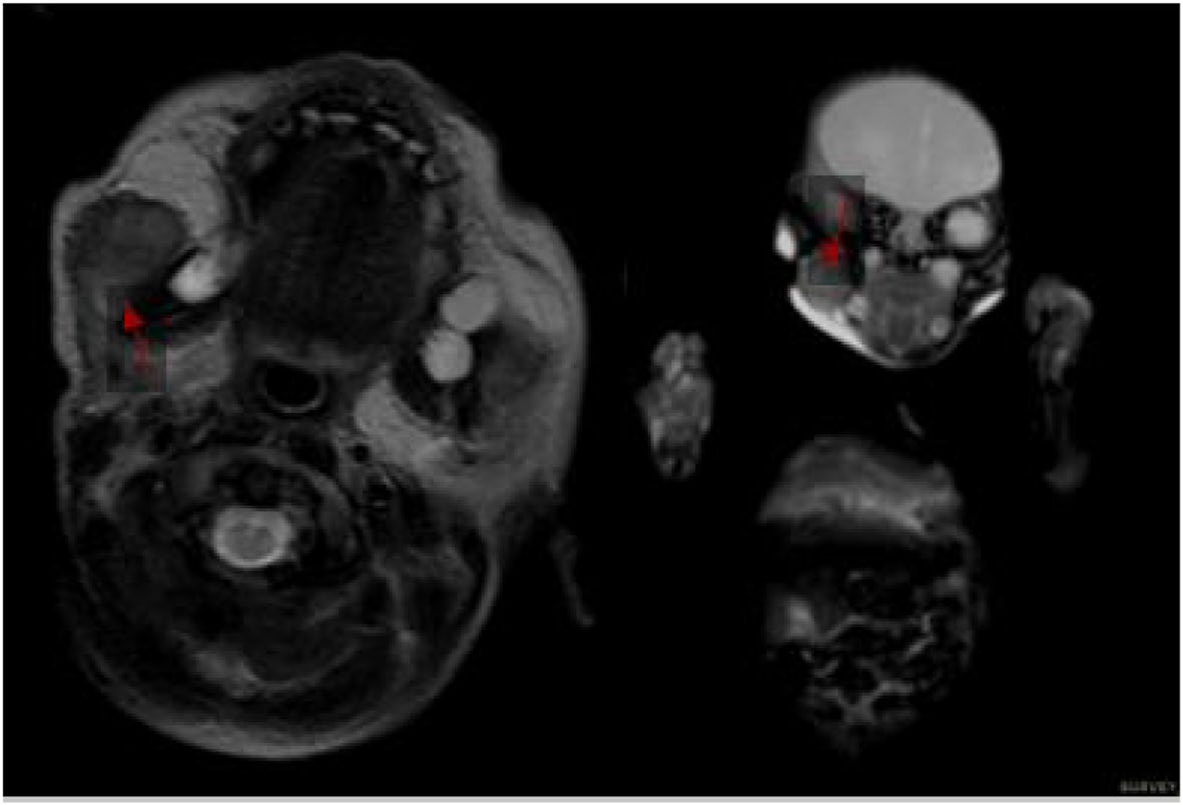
MRI at the age of 20 days: Mandibolar right formation on axial T1 and survey coronal
